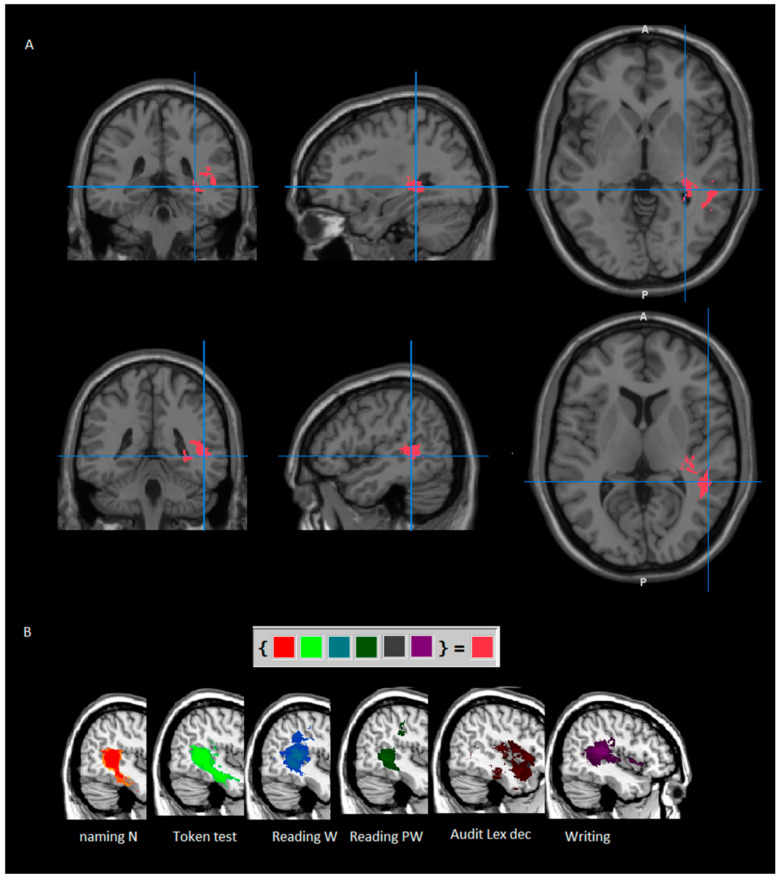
Intersection (A) of lesion masks of the different tasks (B). MR images are displayed following radiological convention (left is right and vice versa). N = nouns; W = words; PW = pseudowords; Audit lex dec = auditory lexical decision.
